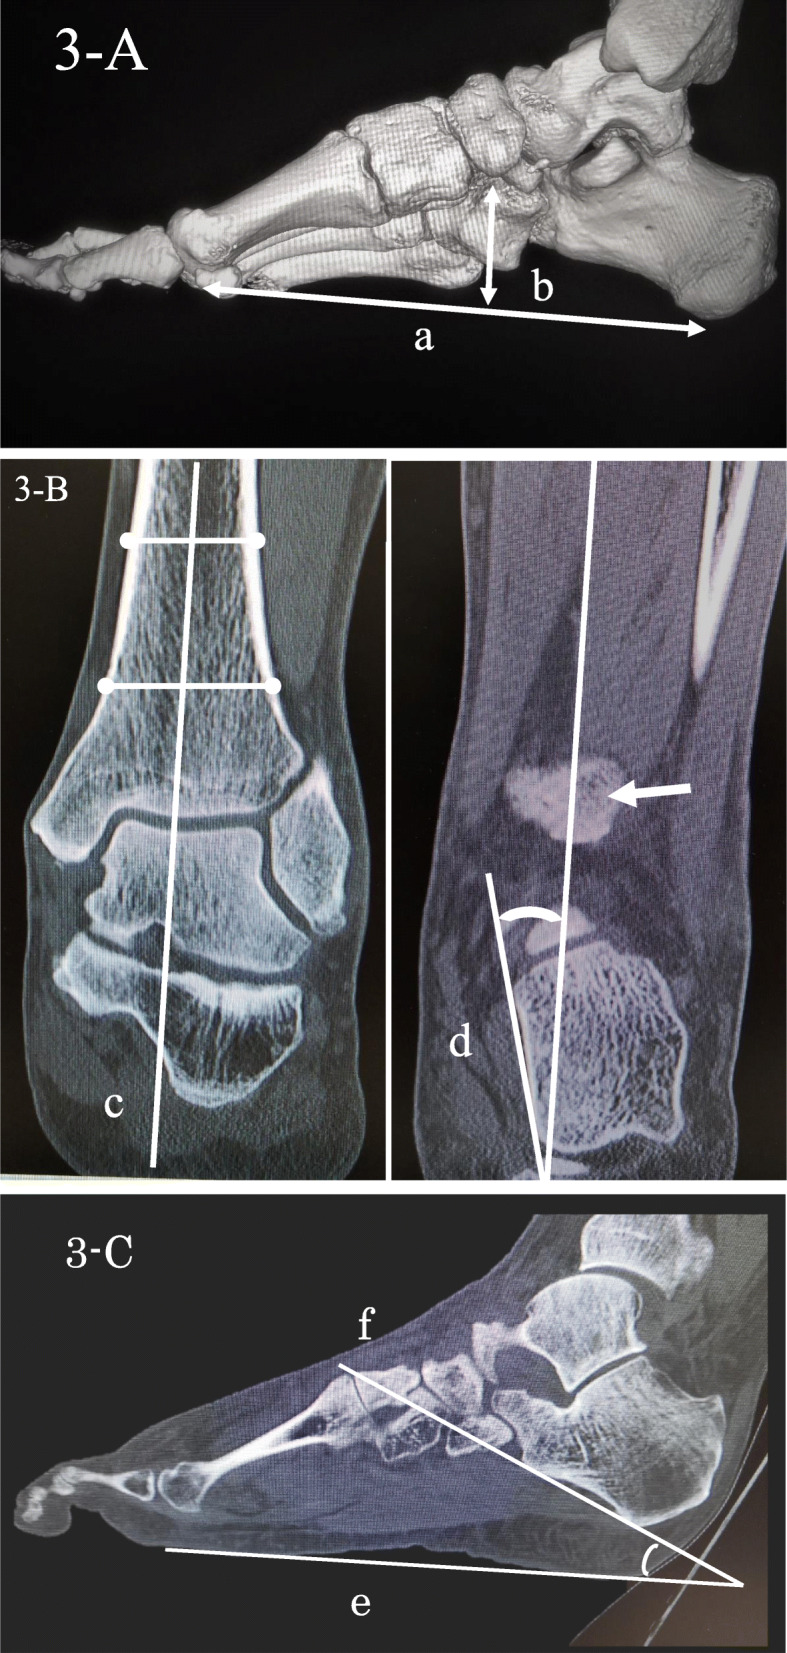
The methodology of measuring the longitudinal arch length and navicular height with 3DCT imaging (A), tibiocalcaneal angle with coronal CT image (B), and calcaneal inclination angle with sagittal CT image (C). A. a: The length of the longitudinal arch. b: The navicular height. B. c: The axis of the distal tibia. d: A line of the medial calcaneal contour. White arrow: The posterior tibial cortex. C. e: The plantar line. f: The most inferior point of the calcaneal tuberosity and the inferior edge of the anterior process of the calcaneus
